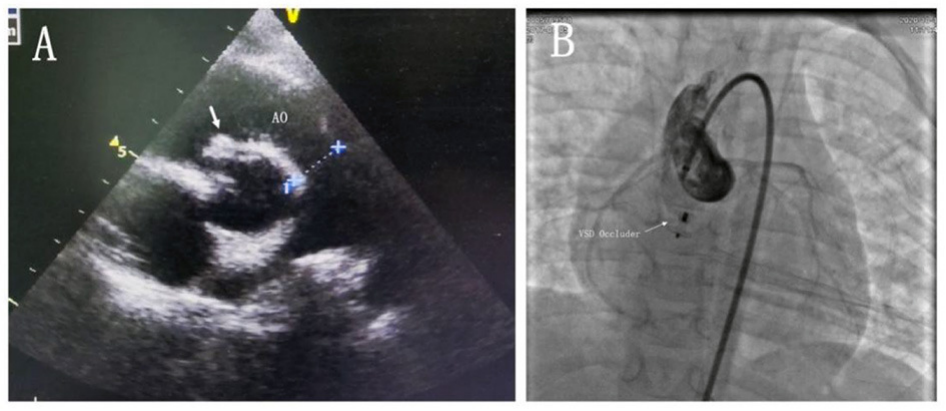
(A) Transthoracic echocardiogram obtained in the aortic long-axis view showing that the occluder was well (white arrow). (B) Cine static image front view showing the ventricular septal defect occluder in place. AO, aorta; VSD occluder, ventricular septal defect occluder.
